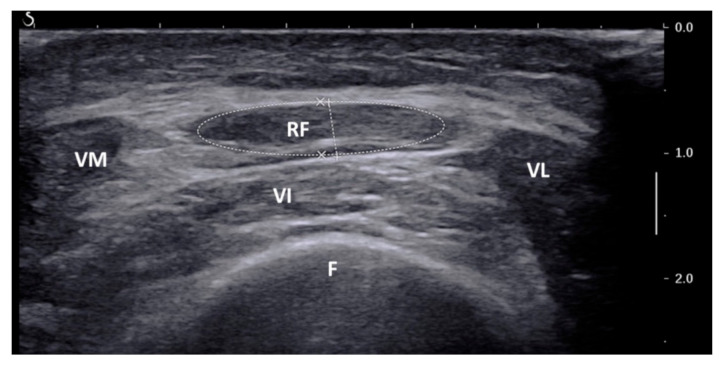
Ultrasound image of the rectus femoris muscle with definition of cross-sectional area (dashed line); RF: rectus femoris; VI: vastus intermedius; VL: vastus lateralis; VM: vastus medialis; F: femur.
